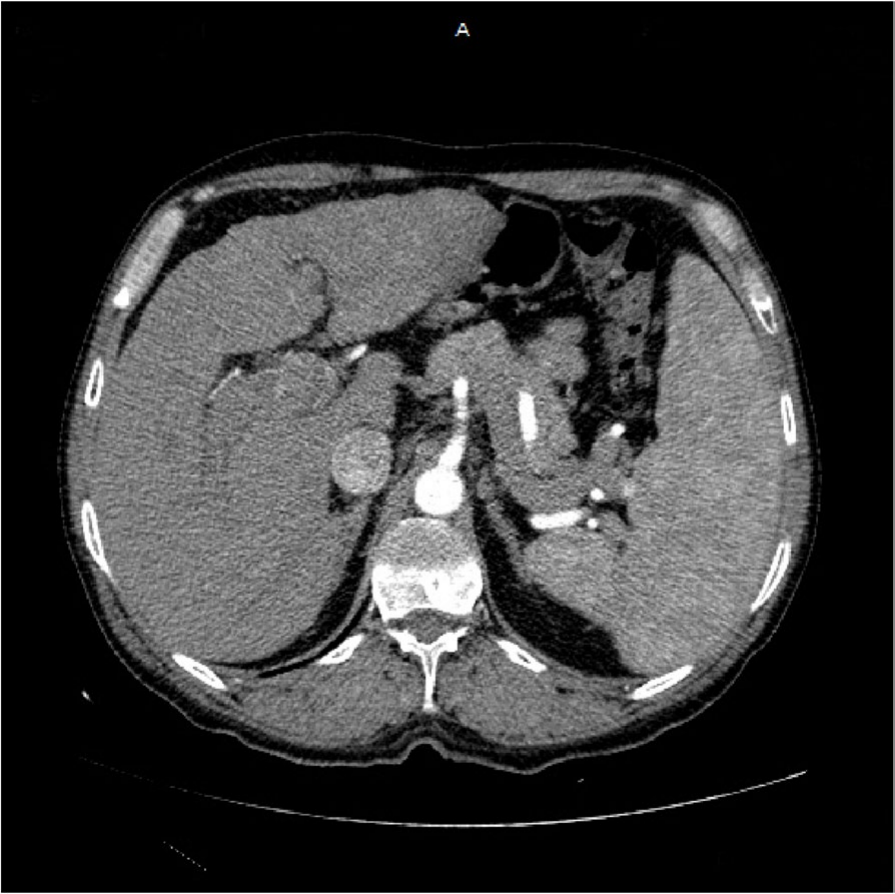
Arterial phase of CT abdomen scan showing fairly defined focal lesion in left lobe segment II measures 13.0 × 7.3 cm show diffuse enhancement
